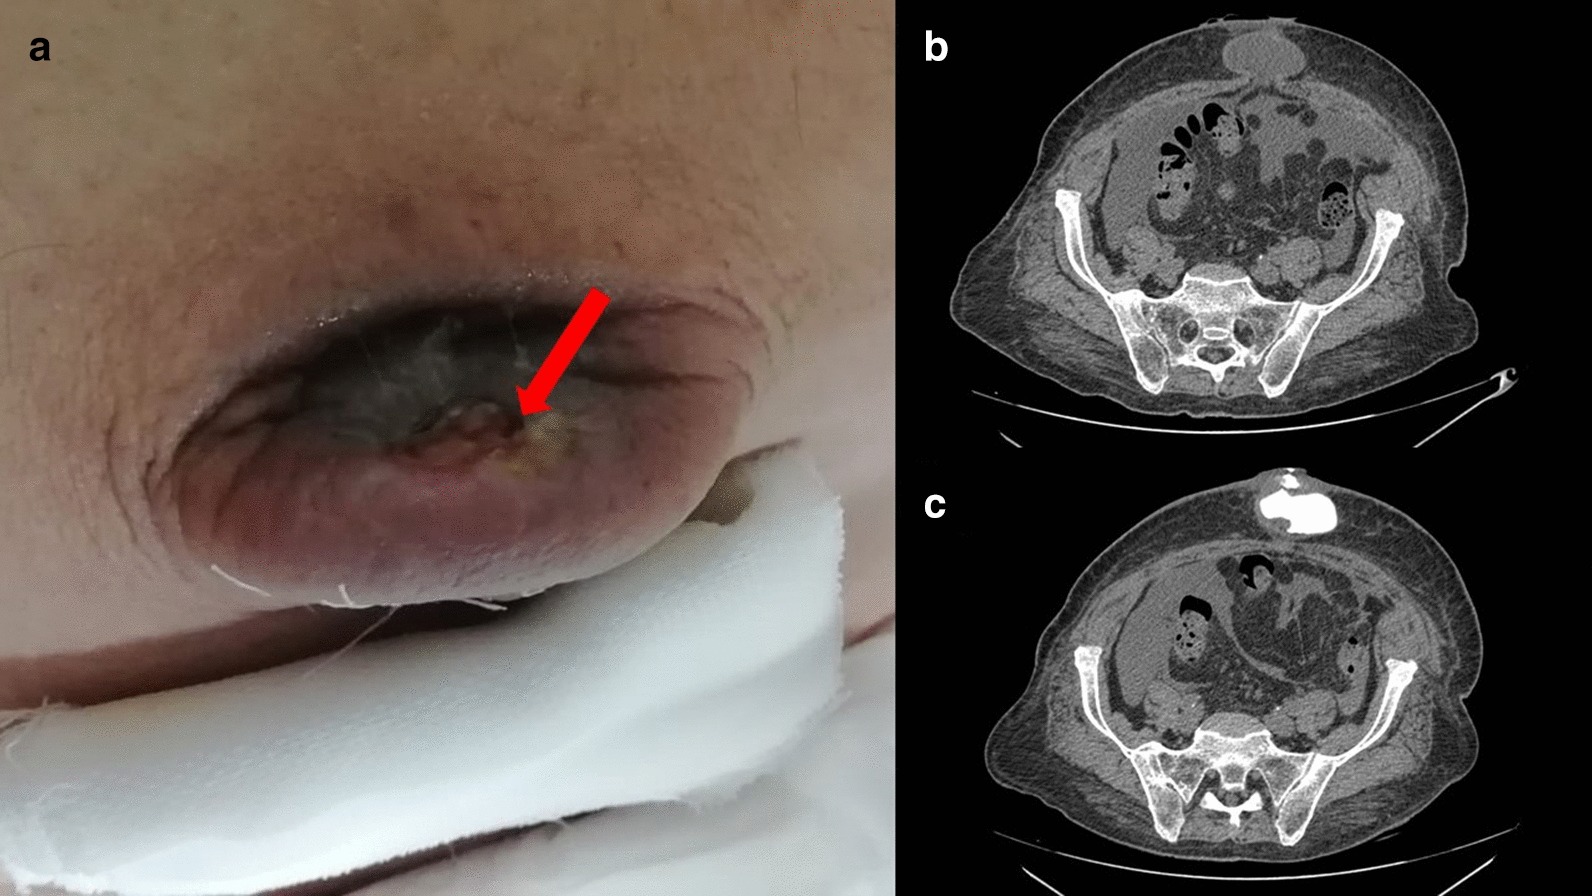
Case 4. a Fistulization of umbilical hernia. Red arrow indicates the cutaneous orifice. b CT-scan showing the umbilical hernia sack with a fluid content. c CT-scan with fistulography showing the diffusion of the contrast agent in the peritoneal cavity from the cutaneous orifice., ruling out intestinal loop fistulization
